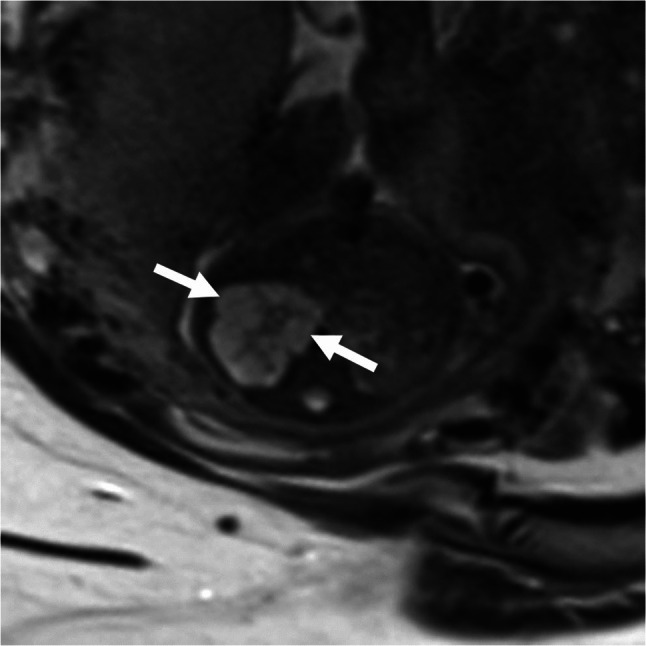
Axial Single-Shot Fast Spin Echo (SSFSE) MRI of a 25-week gestation female fetus with a right multicystic dysplastic kidney. Note the cysts of differing sizes (arrows) replacing the renal parenchyma
